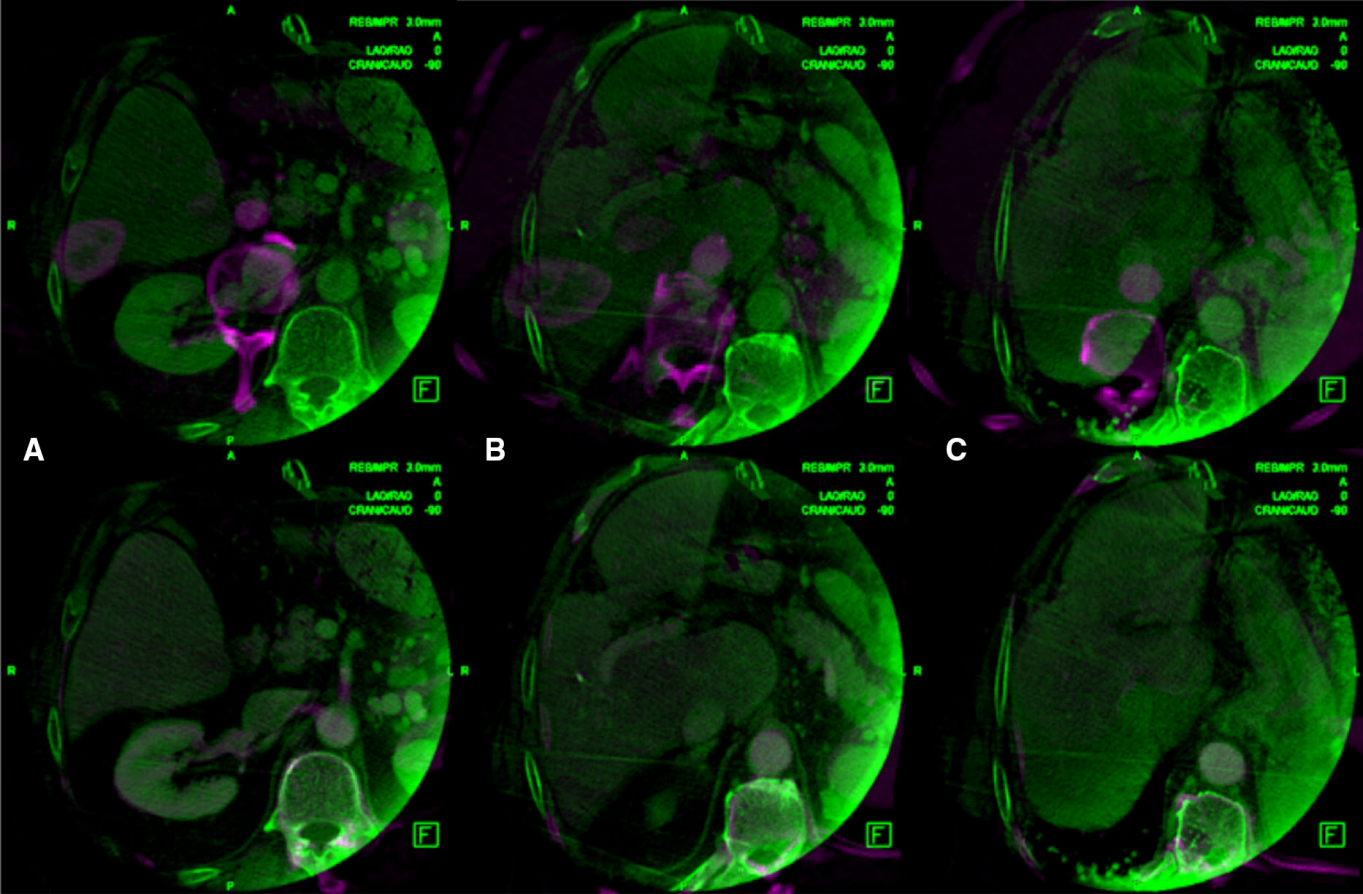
Effects of image registration. Unregistered pre-CT (in purple color) overlapped to CBCT (in green color) (first row) compared to registered pre-CT overlapped to CBCT (second row) for three representative slices of patient #1. The comparison shows that the substantial misalignment occurring before registration (manifest as a purple shadow) was almost completely eliminated using our new fusion method despite the technical differences between the two different imaging modalities
